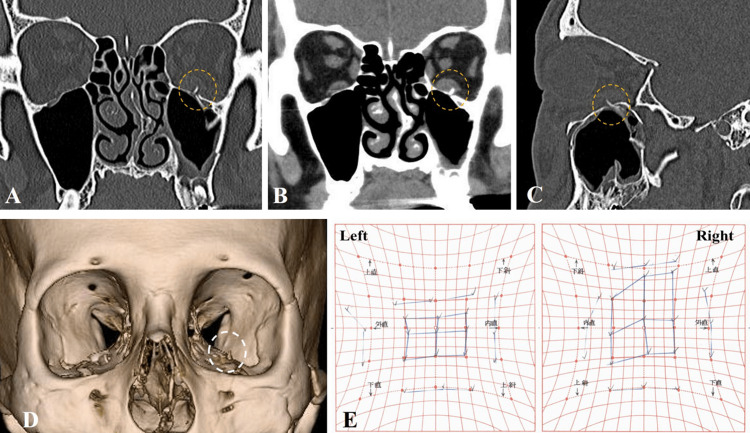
Preoperative CT and Hess charts. Images five days after the injury. Bone window coronal CT (A), soft tissue window coronal CT (B), bone window sagittal CT (C), and 3D-reconstruction CT (D) show a linear fracture of the left orbital floor and the protrusion of a small bone fragment into the orbital space (dot circle). (E) Hess charts showing the significant limitation of left eye movement in the upper gaze.
